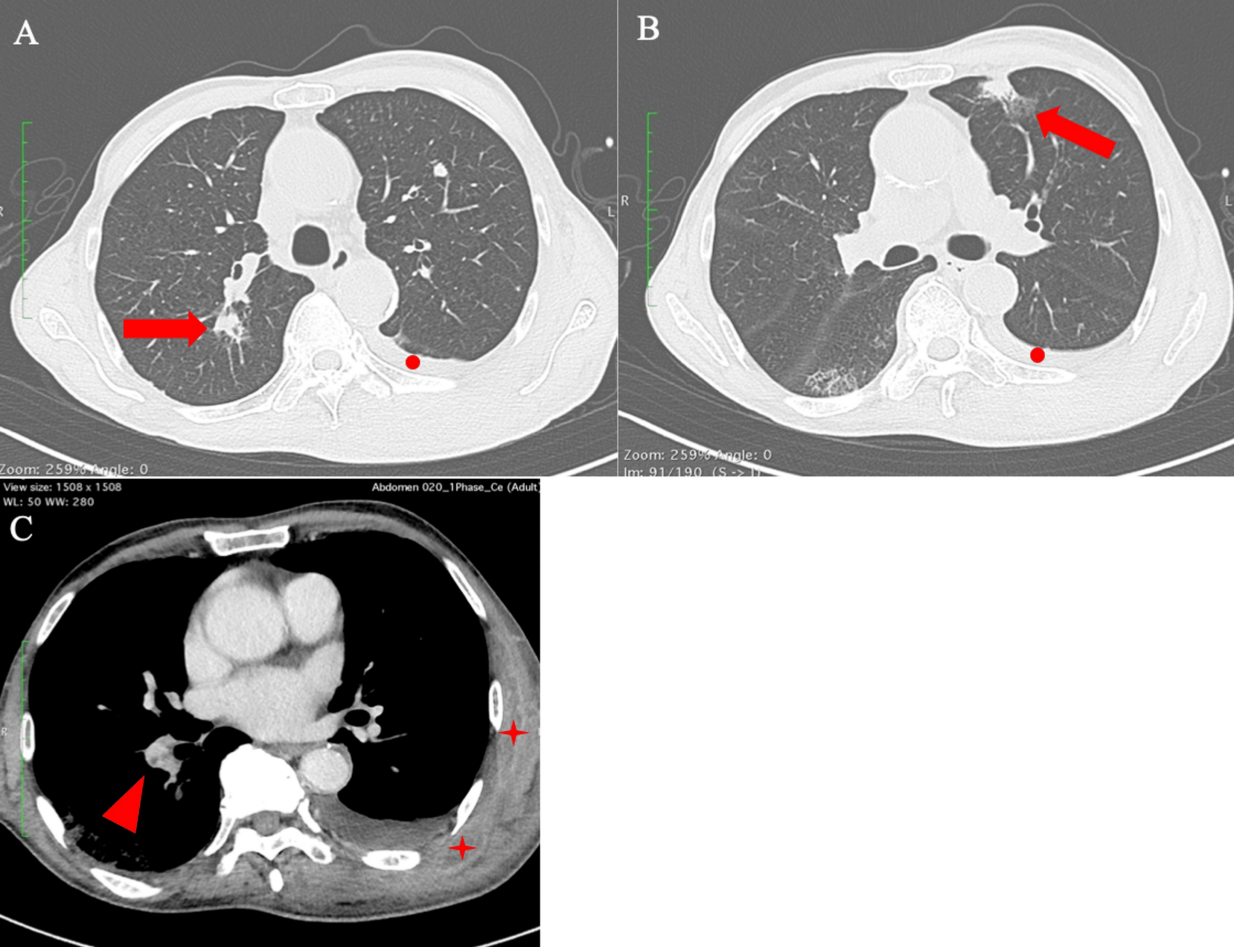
Chest contrast-enhanced computed tomography A and B: Pleural effusion in the left lung (red circles) and lung nodules in both lungs (red arrows). C: Pulmonary embolism in the right pulmonary artery (red arrowhead) and intramuscular hematomas in the left back (red stars).
